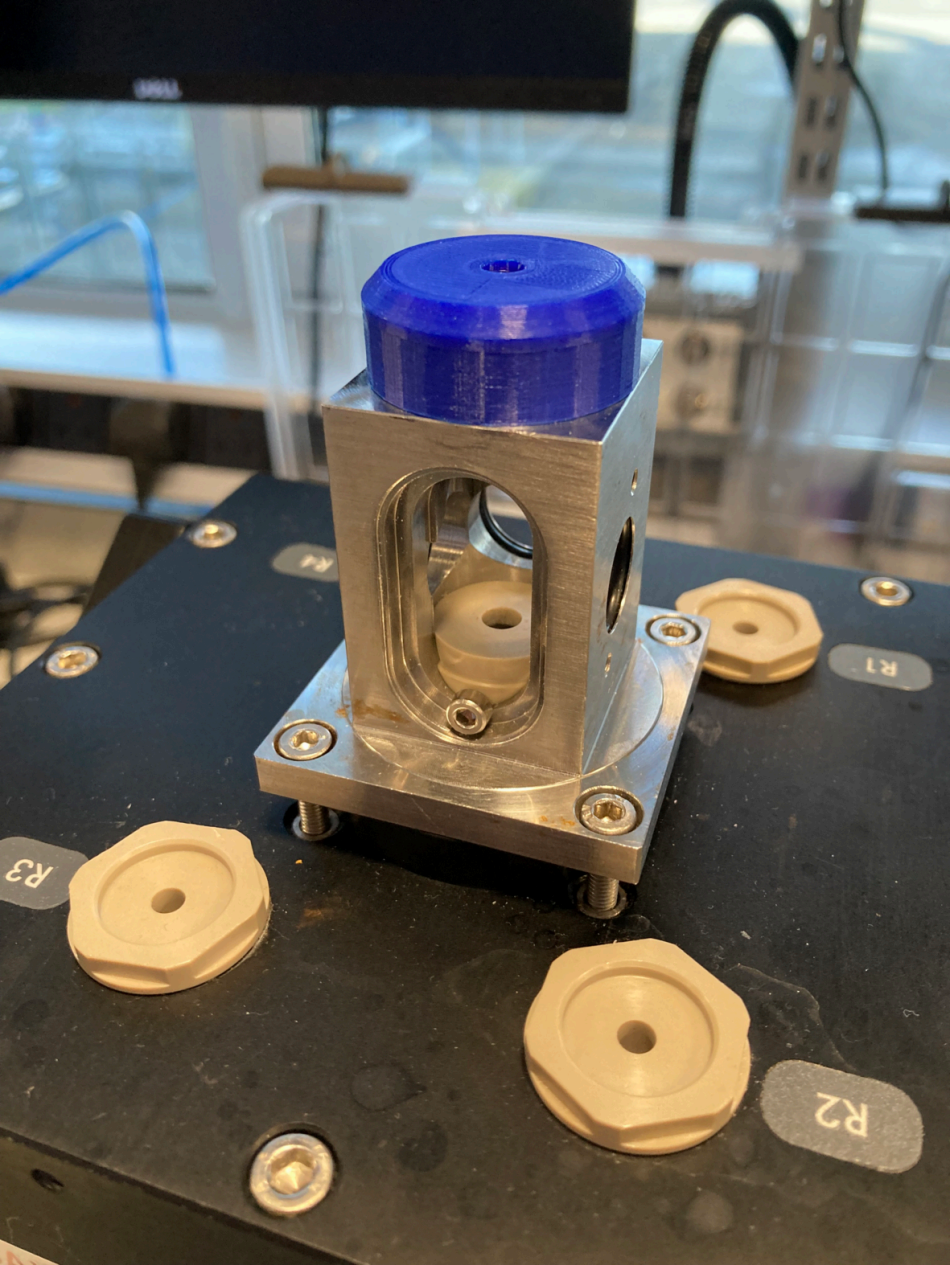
The X-ray head installed on the stop-flow unit. The blue compression screw top forces the tip of the capillary holder (Fig. 2 ▸) inside the head into an o-ring seal in the base of the unit. This compression also pushes a second o-ring seal into the glass capillary. The blue screw top in this image is a 3D printed replacement for the manufacturer’s part which perished due to exposure to solvents. STL files can be found in the supporting information.
